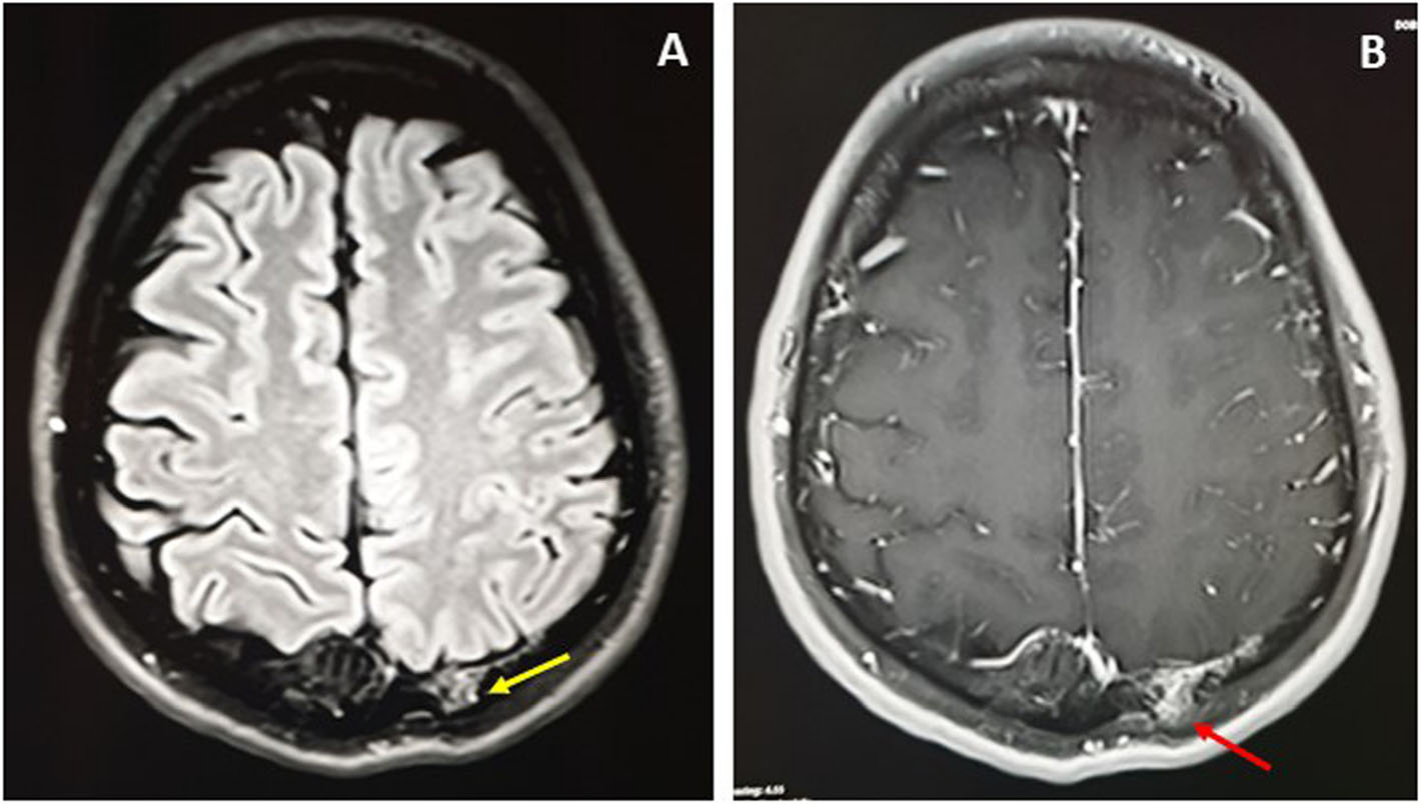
Fluid attenuated inversion recovery (FLAIR) MRI (a), and post contrast T1 (b) show the left parietal diploic lesion with external wall erosion (Yellow arrow) and subgaleal soft tissue enhancement (Red arrow)
